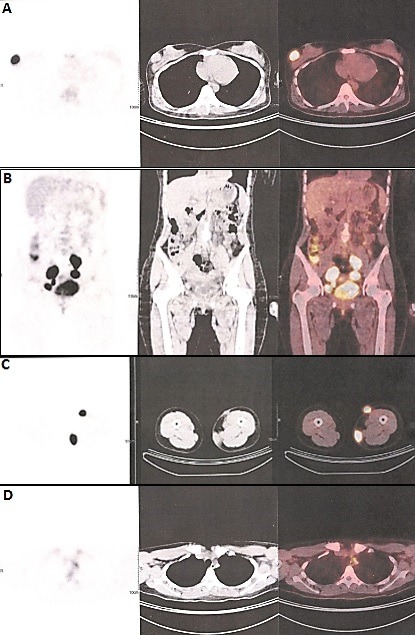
TEP-TDM d’évaluation thérapeutique: (A) une augmentation en volume et en intensité du nodule sous cutané du sein droit (SUVmax à 11,87 vs 6,55); (B) un magma d'adénopathies supravésical latéralisé à gauche et latérovésical droit (SUVmax respectif à 17,91 et 16,69) avec l'apparition d'adénopathies inguinales profondes; (C) une augmentation en volume et en intensité des nodules cutanés de la face antérointerne et interne de la cuisse gauche (SUVmax respectif à 12,64 et 15,37); (D) une adénopathie infracentimétrique latérotrachéale gauche de SUVmax à 3,58
